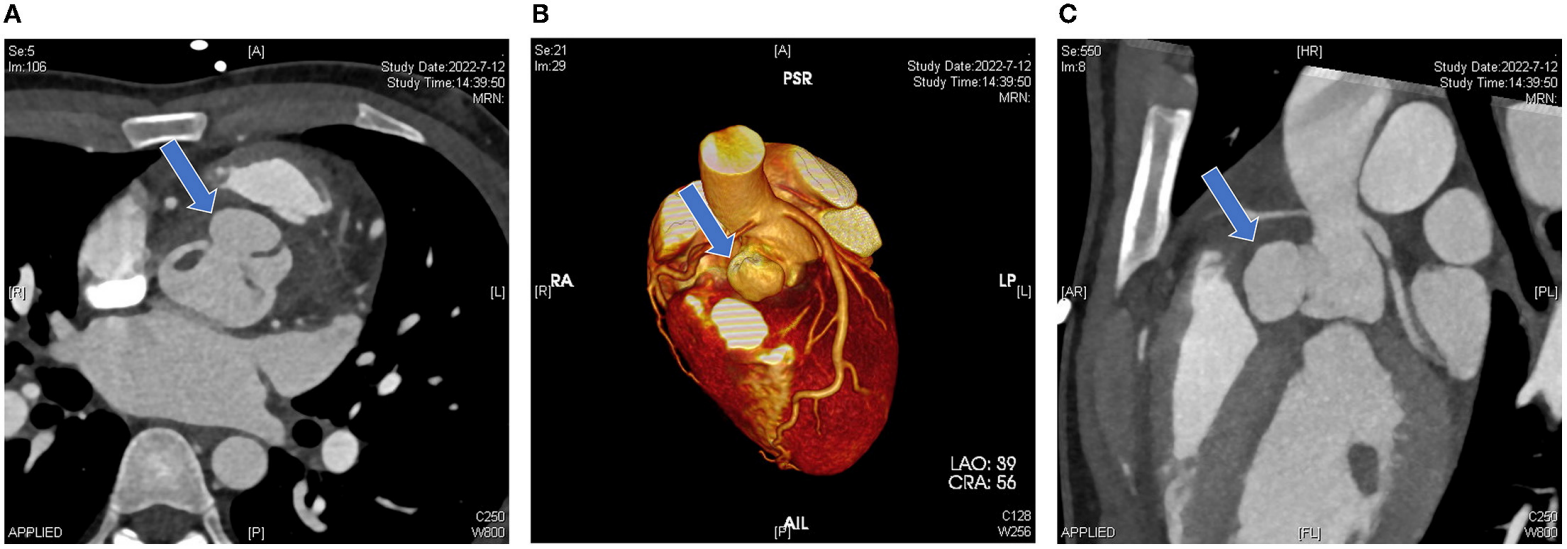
Pre-operative computed tomography. (A) Irregular morphology of the coronary sinuses with local anterior protrusion (blue arrow). (B) A three-dimensional reconstruction image shows that the left coronary artery arises from above the left coronary sinus and the right coronary artery arises from above the junction of the left and right coronary sinuses. The aortic root bulge is shown in the blue arrow. (C) Sagittal view showing the vertical position of the bulge (blue arrow).
